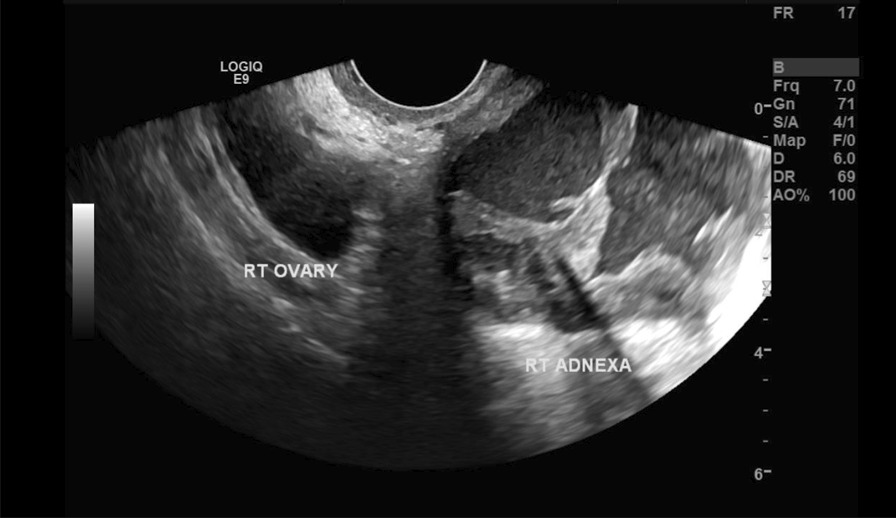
Ultrasound reveals right sided mass. The mass appears irregularly lobulated and heterogeneous measuring 4.5 × 4.3 × 4.3 cm in size
